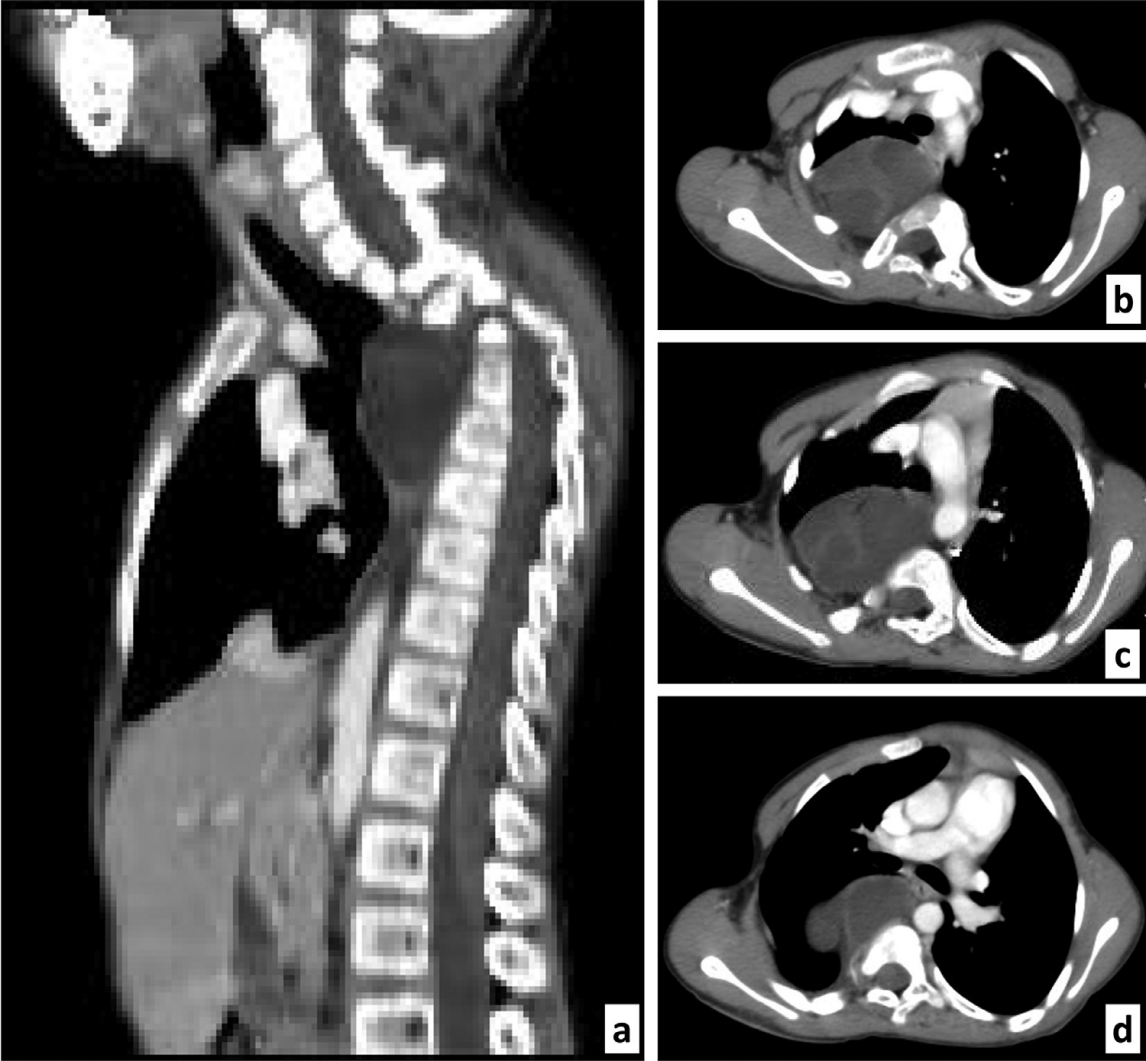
Contrast-enhanced chest CT scan, mediastinal window. Both the midsagittal slice (A) and the 2 axial transpedicular slices at T3 (B,C) and T4 vertebrae (D) demonstrated the T3 right pedicle defect that made a way to an extruded inhomogeneous mass (signals iso- to hypodense to the parietal muscles). Note the right shifted dural sac (B,C,D).
